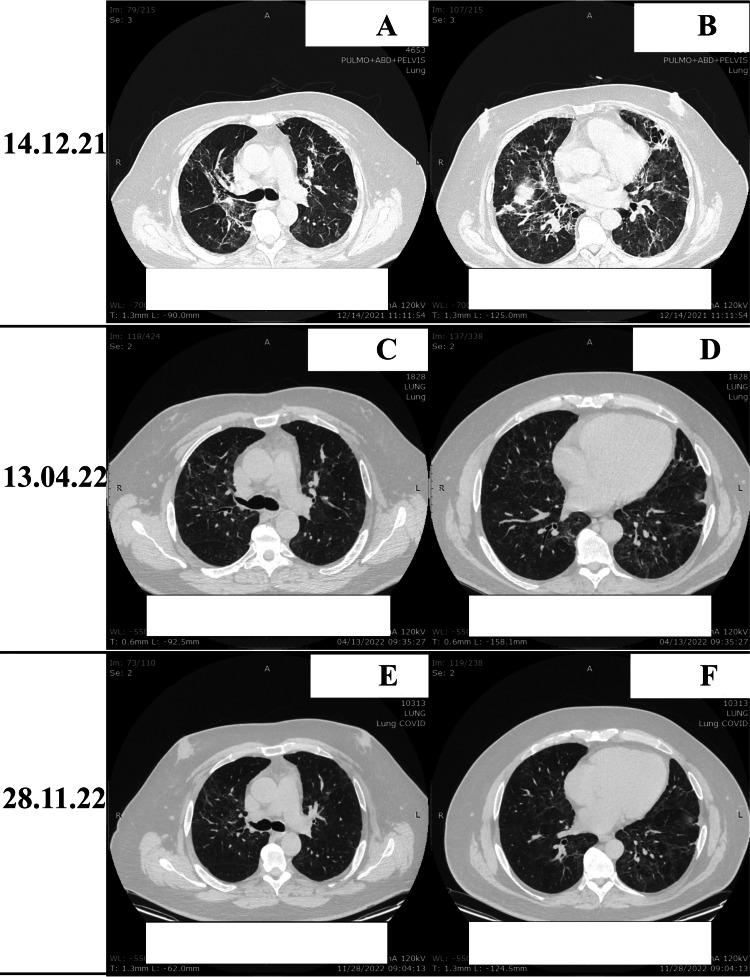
Control CT of lung А,B) Gross reticular changes with single and areas of consolidation bilaterally in the parenchyma; C,D) Still persistent reticular changes, single areas of consolidation peripherally; E,F) Reduction in the area of post-inflammatory changes associated with SARS-CoV-2.
